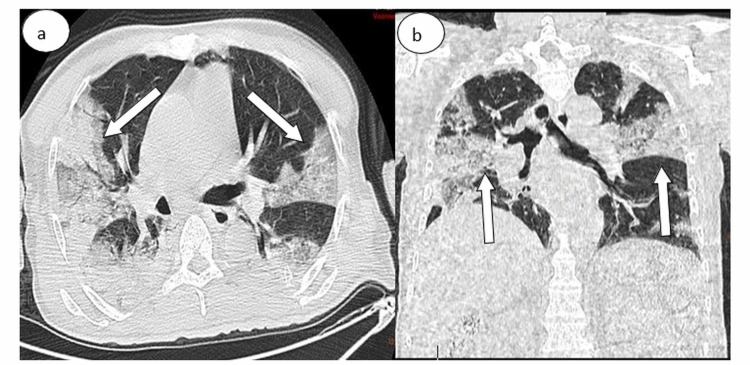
Thoracic CT scan in (a) axial and (b) coronal lung windows showing some ground-glass opacities (white arrows) associated with a bilateral massive consolidation The percentage of lung involvement is approximately 50%.
